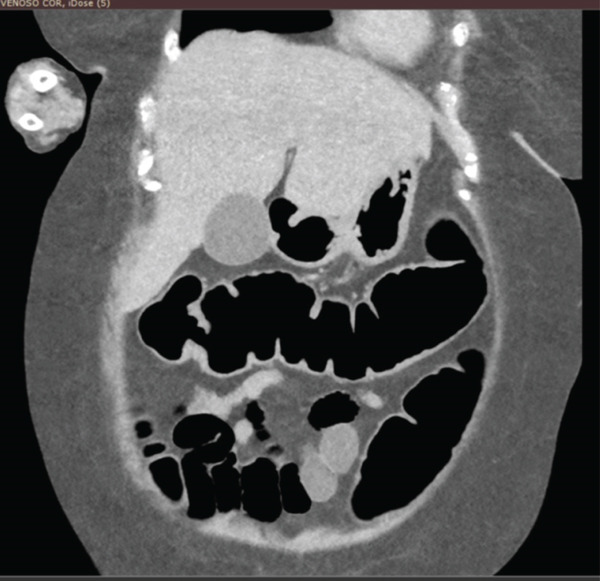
Computed tomography of the abdomen (coronal projection) demonstrating extensive bowel distention.
